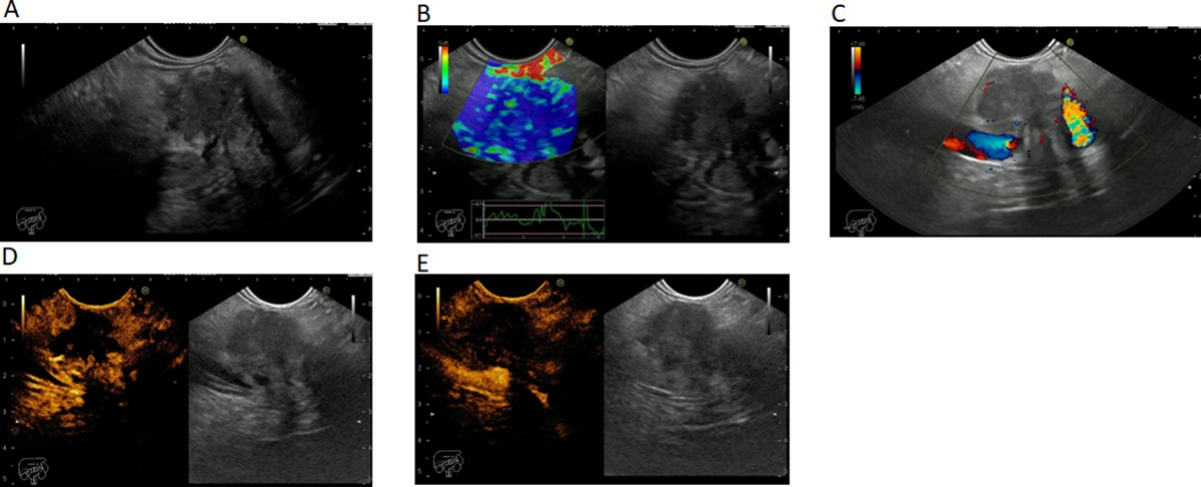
EUS imaging of a pancreatic ductal adenocarcinoma in (A) gray scale. (B) elastography. (C) color Doppler. (D) contrast enhancement–arterial phase. (E) contrast enhancement–venous phase.
